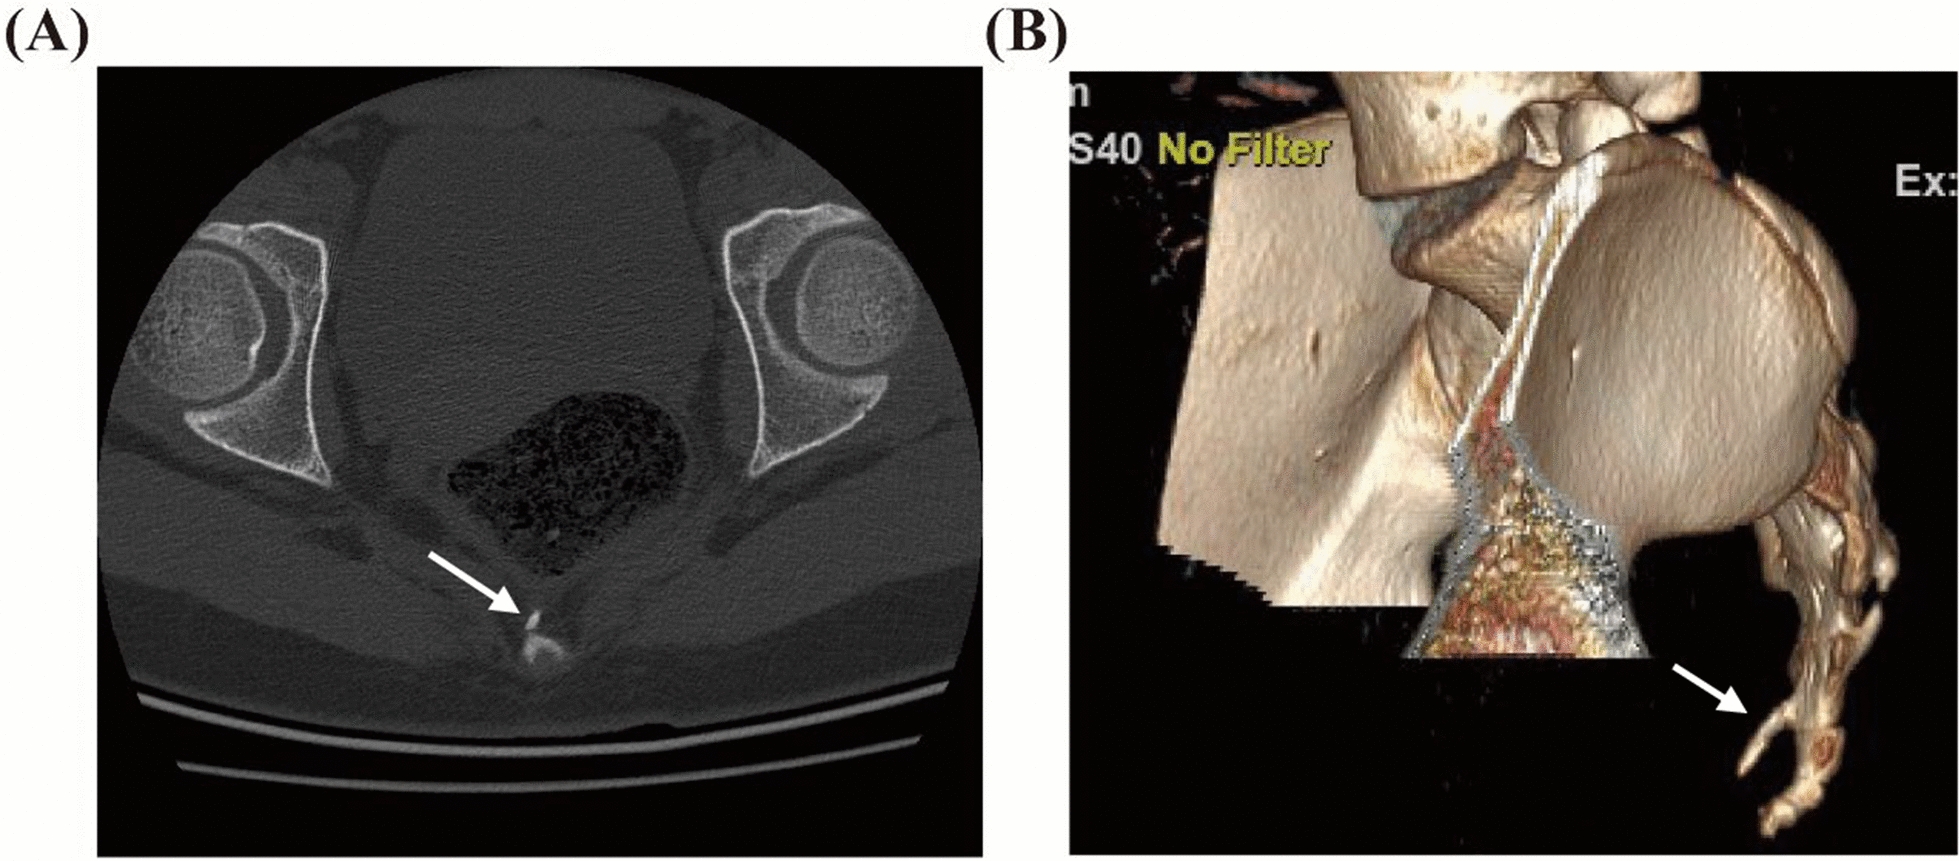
A Computed tomography of the sacrum and coccyx with B three-dimensional reconstruction; the area indicated by the arrow is the abnormal skeletal structure emanating from the first caudal vertebra
